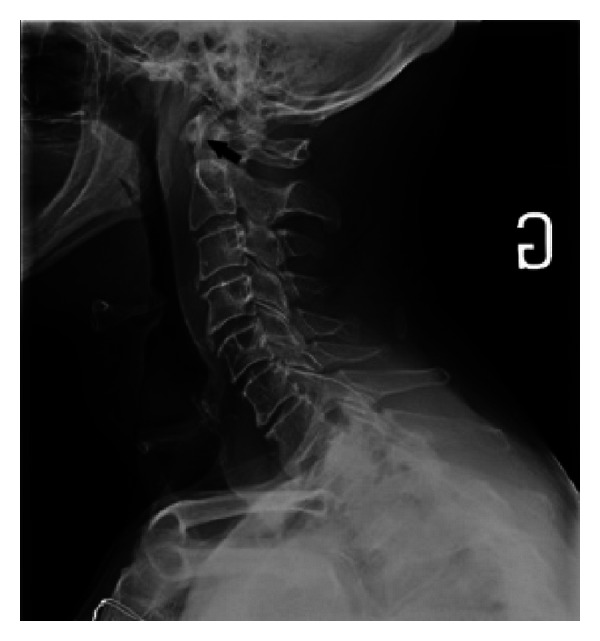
On the radiography of the neck, note the presence of an ill-defined calcification at the level of C1 (black arrow).
